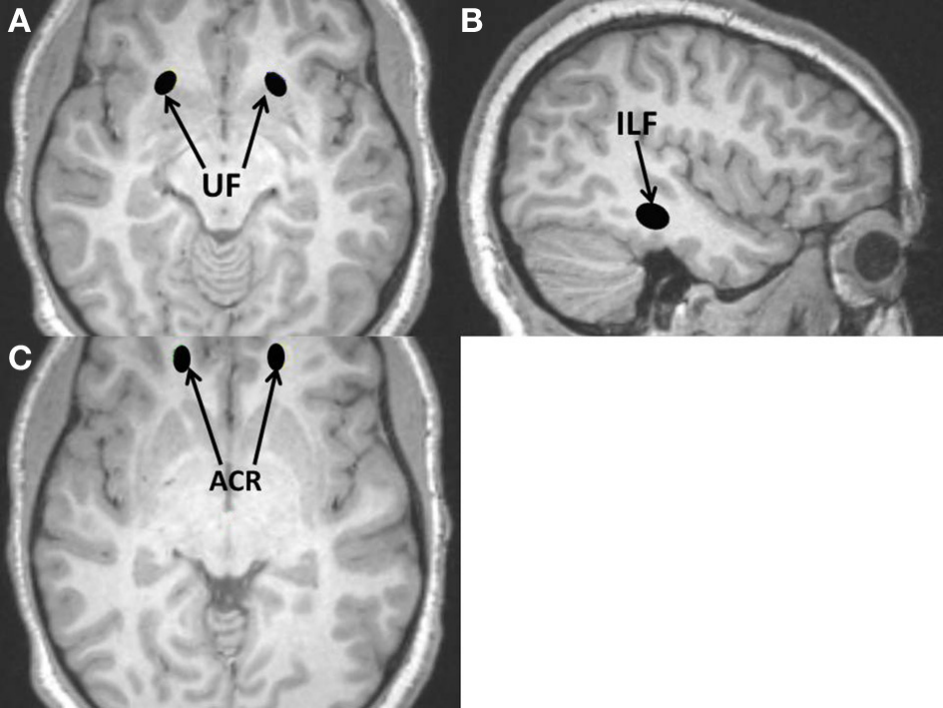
Additional regions of interest (ROIs) used for DTI measurements placed on anatomical T1-weighted MRI scan; all are non-auditory ROIs. (A) Uncinate fasciculus (UF); (B) Inferior longitudinal fasciculus (ILF); (C) Anterior corona radiata (ACR).
